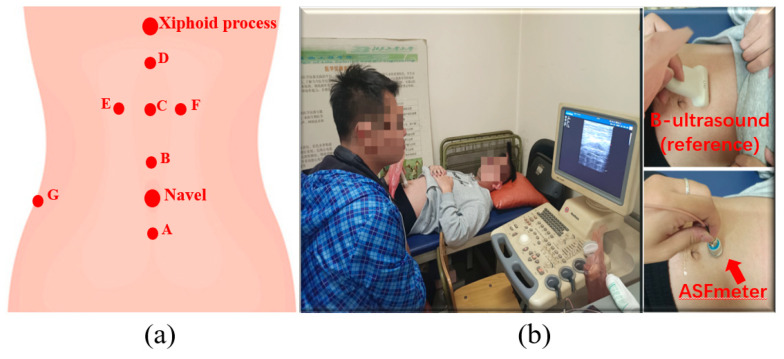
ASF thickness measurement: (a) The measurement positions on the abdomen. (b) Measurement using the ASFmeter and a B-mode ultrasound device.
